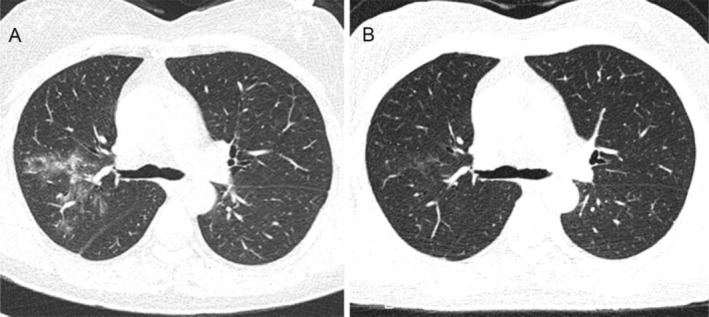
(A) Chest CT showing multiple patchy lesions appeared in the bilateral lungs, especially in the right upper lung. (B) Patchy shadows in the right lung dissipated after 1 week of treatment.
